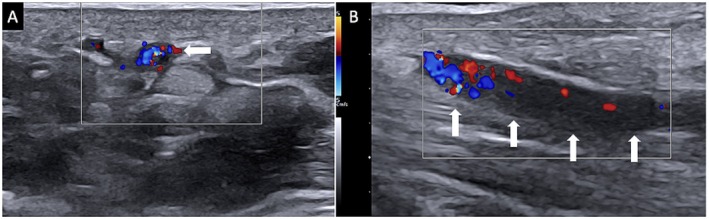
Transverse (A) and longitudinal (B) gray‐scale ultrasound images demonstrate a dilated hypoechoic tubular mass with internal echogenic material and lack of normal arterial flow at the level of the carpal tunnel (white arrows).
